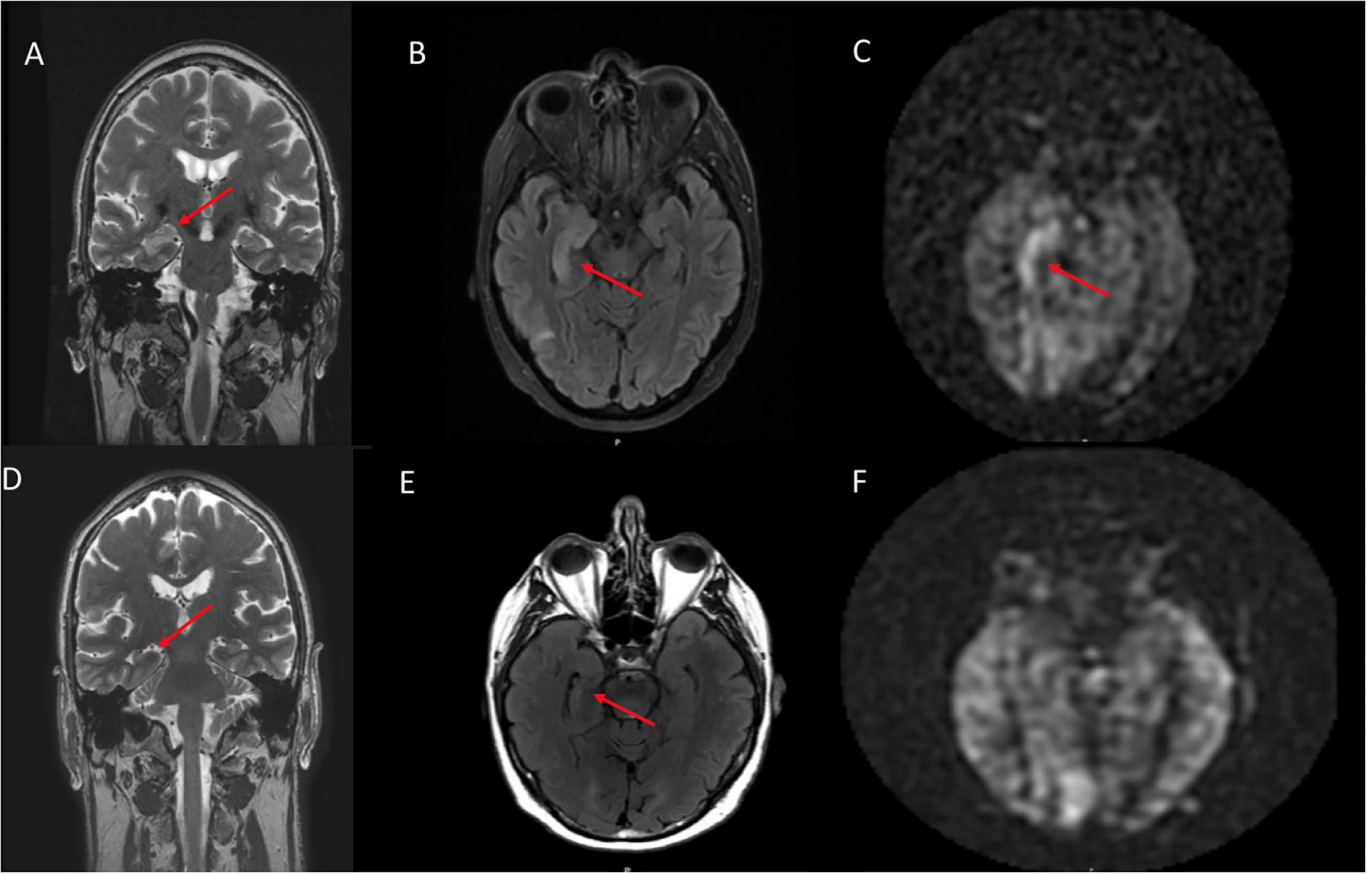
A 70 year old male who presented with rapid cognitive decline and spasmic extremity movement. (A) Initial coronal T2W shows asymmetric T2 hyperintense signal in the right hippocampus. (B) Axial FLAIR demonstrates asymmetric hyperintense signal in the right hippocampus and focal cortical signal abnormality in the posterior right temporal lobe. (C) ASL shows focal hyperperfusion in the right hippocampus and milder asymmetric signal in the posterior right temporal and right occipital lobes. (D) 12 month follow up coronal T2W shows mild right hippocampal volume loss. (E) Follow up axial FLAIR shows mild right hippocampal volume loss and hyperintense signal. (F) Follow up ASL shows complete resolution of the previously right hippocampal hyperperfusion. Of note, the decreased ASL signal in the left occipital lobe is due to the present of an ipsilateral fetal PCA.
